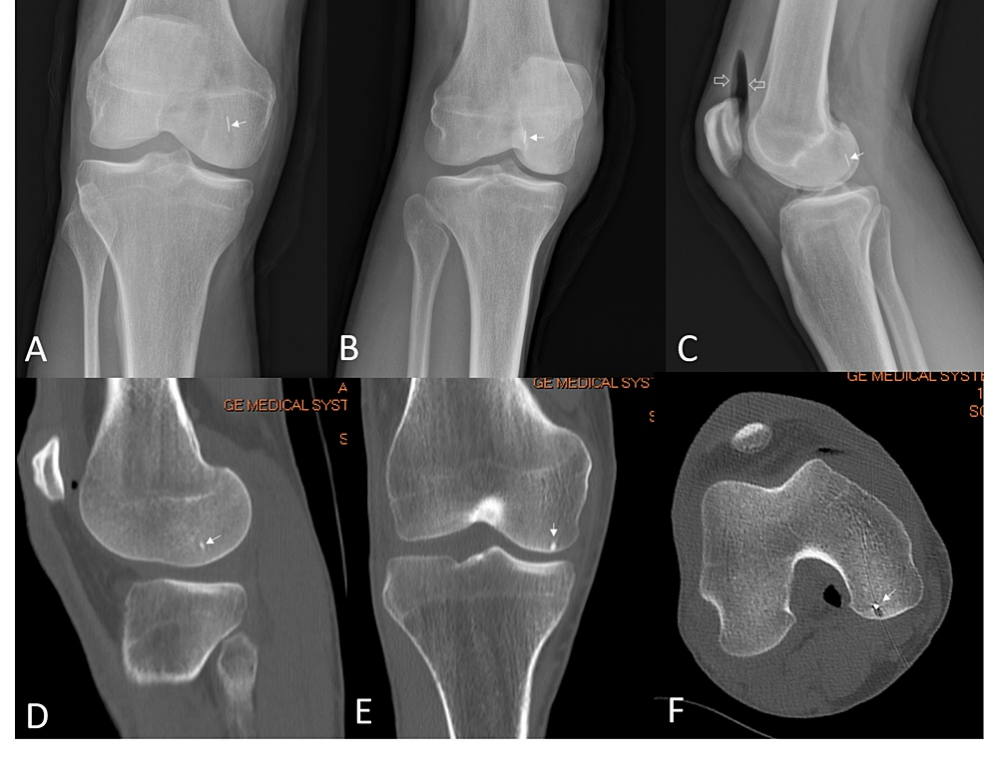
Post-nail removal X-rays (A-C) and CT scan images (D-F). Lateral view (C) showing intra-articular air (open arrows) as a result of irrigation.
